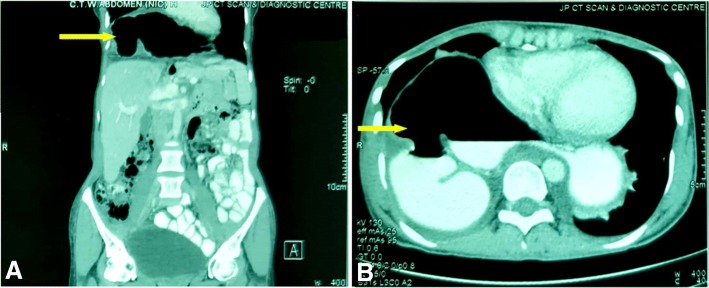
Preoperative coronal (a) and axial (b) computed tomography slice showing gastric bubble (yellow arrow) indicating herniation of stomach into the thorax
